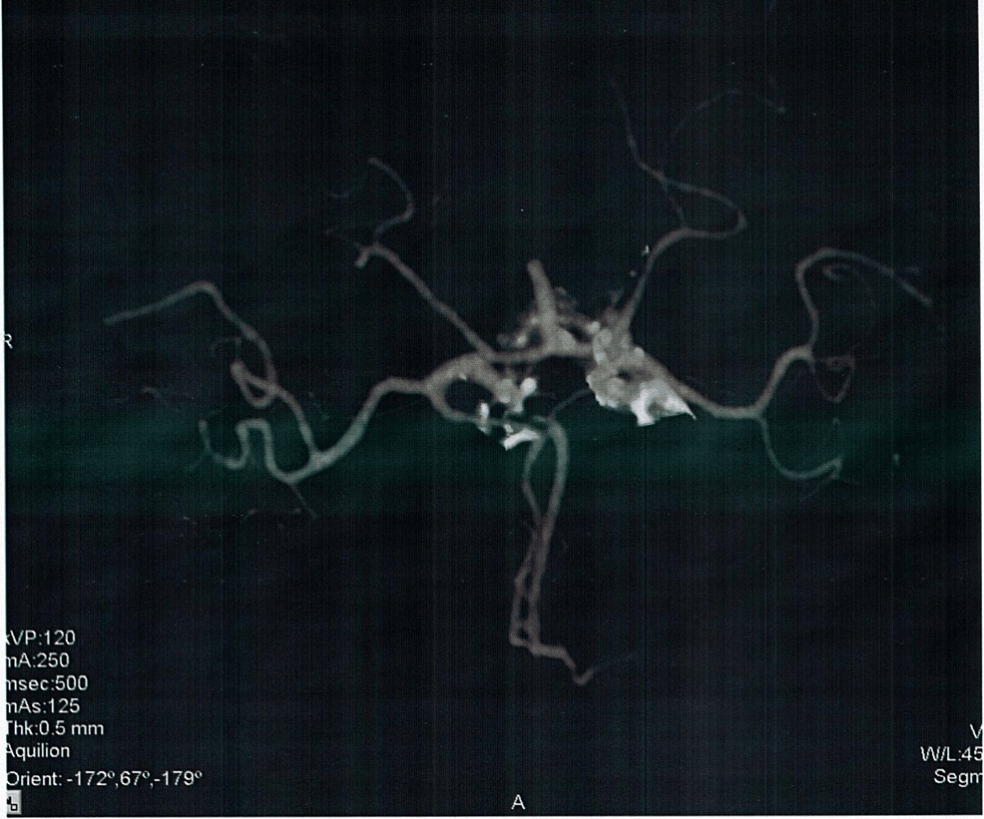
Computed tomography angiogram depicting hyper-densities
